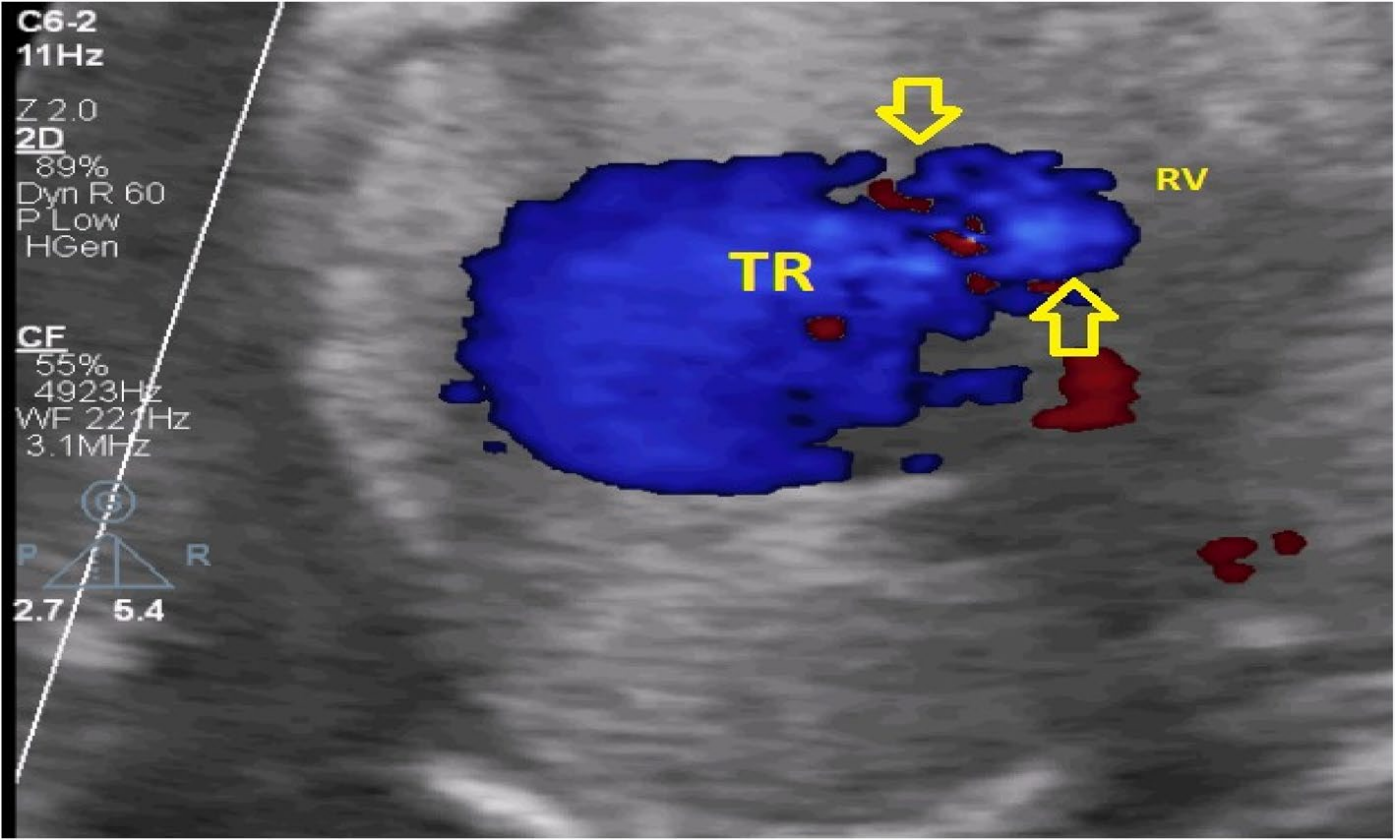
Color Doppler shows severe tricuspid regurgitation (TR), yellow arrows. RV: right ventricle
